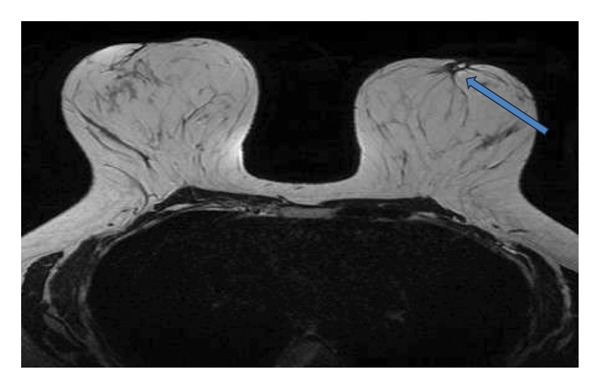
The patient (the same as in Figures 8, 9, 10) underwent ce‐MRI examination. The T2‐weighted sequence shows a small left retro areolar fluid collection, associated with distortion of the adjacent breast parenchyma (arrow in 11).
